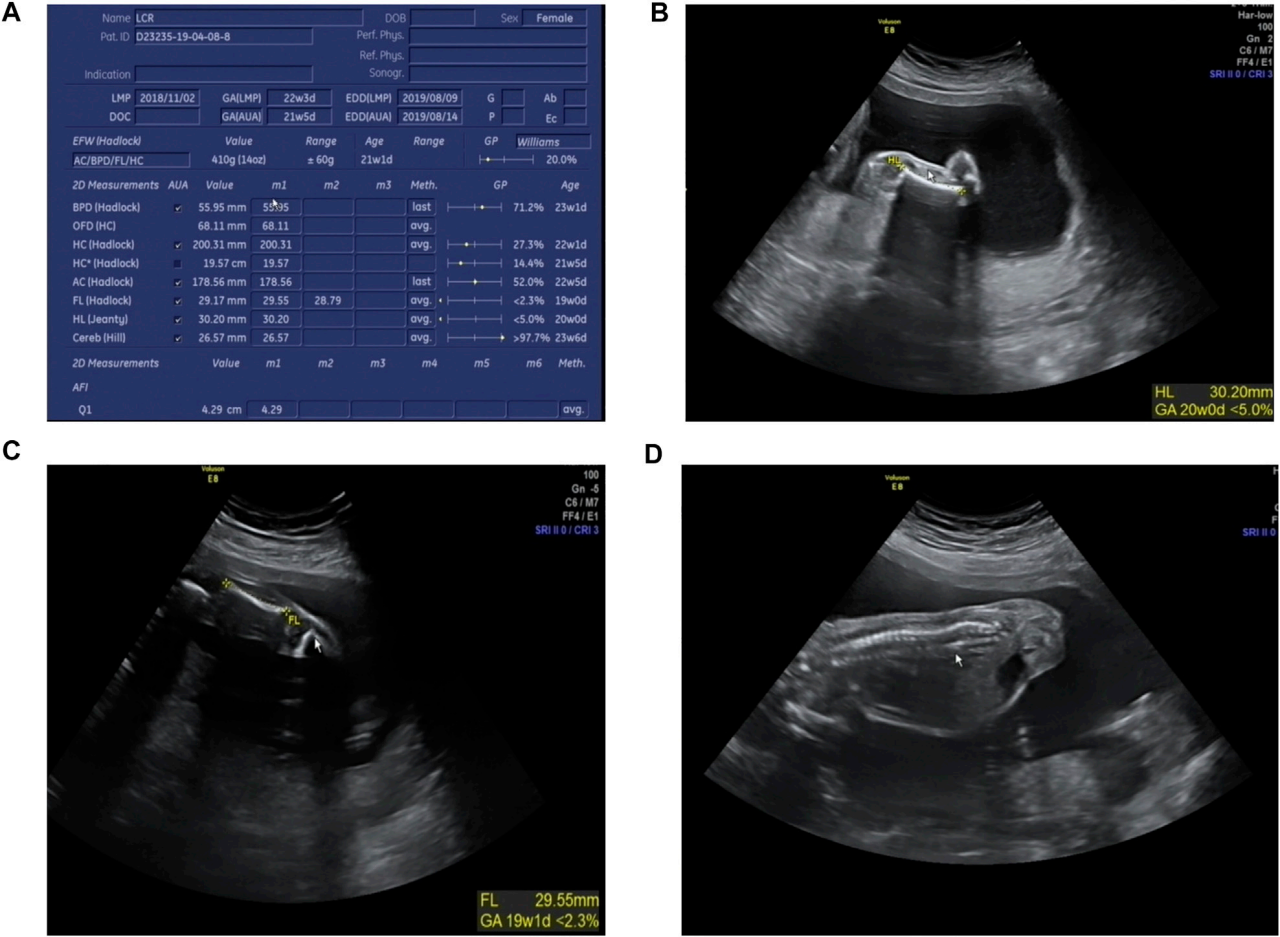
Radiographic features of the fetus (at 22^+3^ weeks’ gestation). (A) The fetus was generally smaller than the fetus at the same gestational age; (B) HL was equivalent to that of fetus at 20 weeks gestation; (C) FL was equivalent to that of fetus at 19^+1^ weeks’ gestation; (D) Fetus scoliosis could be seen.
